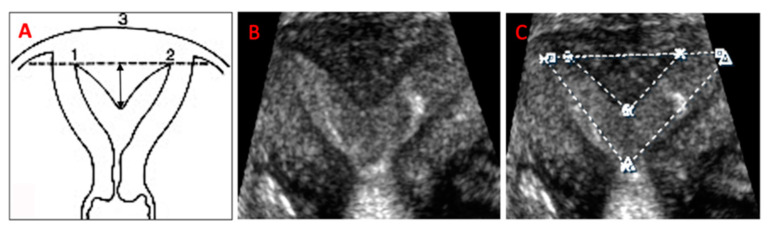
(A). Graphic representation and 3-D ultrasound measurement technique of uterine subseptations (1,2= left and right tubal ostia; 3= fundal outer contour; arrows: measurement of subseptation length). (B). 3-D ultrasound coronal view rendering of a subseptation. (C). Measurement of the cavity width (1–2), and subseptation width, for calculation of cavity area on the 3-D ultrasound coronal view.
